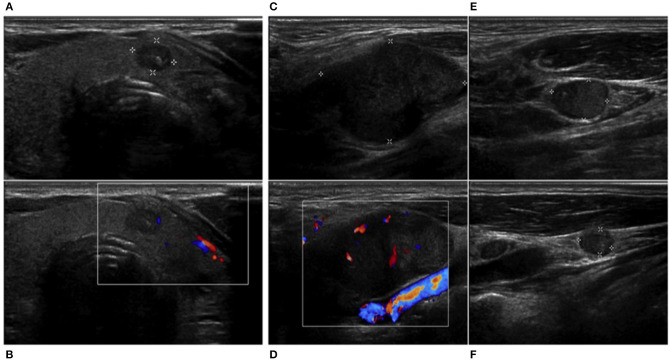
Thyroid and neck ultrasound. An irregular hypoechoic nodule (11 × 7 mm) with (A,B) calcifications in the paraisthmic portion of the left thyroid lobe and (C,E,F) multiple suspected lateral cervical rounded, hypoechoic, and inhomogeneous lymphadenopathies without ilum, showing an panel (D) unorganized peripheral and central vascularization.
